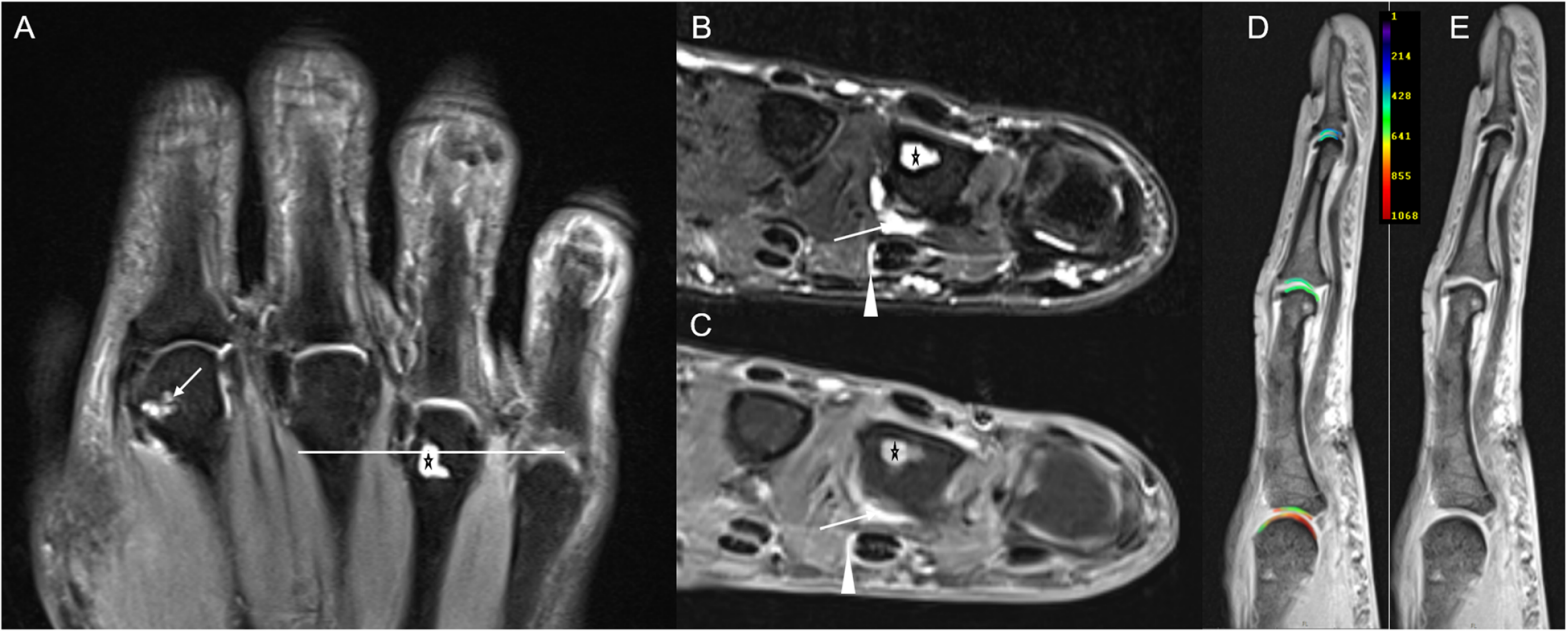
Right hand of a 57-year-old female with PsA (disease duration 46 months). Coronal STIR of digits 2–4 (a), transversal T2w fs (b), and corresponding contrast-enhanced T1w fs images (c) of the metacarpophalangeal (MCP) level of digits 2–4. The horizontal white bar in a indicates the level of transversal slices (b, c). a Bone erosions at the metacarpal head of the 2nd (arrow) and 4th (asterisk) digits. b, c Bone erosion at the fourth metacarpal head (asterisk), synovitis (arrow), and subtle flexor tenosynovitis (arrowhead) at the MCP joint level of the 4th digit. d, e Representative sagittal T1-weighted images of the MCP, PIP and DIP joints of the 3rd digit. Following iv contrast administration and appropriate delay of 40 min, d gives the morphological T1 map, while e gives the corresponding parameter map with dGEMRIC values [ms] overlaid. No marked decrease of dGEMRIC indices is seen
